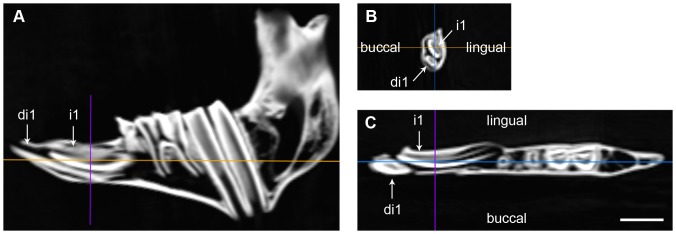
CT-scan images of the mandible IMEDEA 48059 of *M. balearicus*. Replacement of the deciduous first incisor by the permanent one. (*A*) Sagittal plane. (*B*) Coronal plane. (*C*) Transversal plane. Scale bar  = 1 cm.
